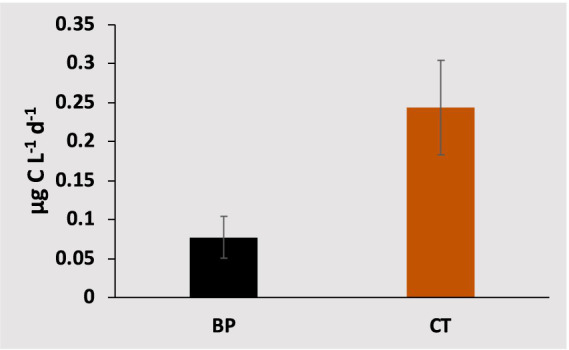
Average values (± standard error) for bacterial carbon production (BP, black bar) and carbon transferred to the food web (CT, orange bar), measured from the same incubations.
